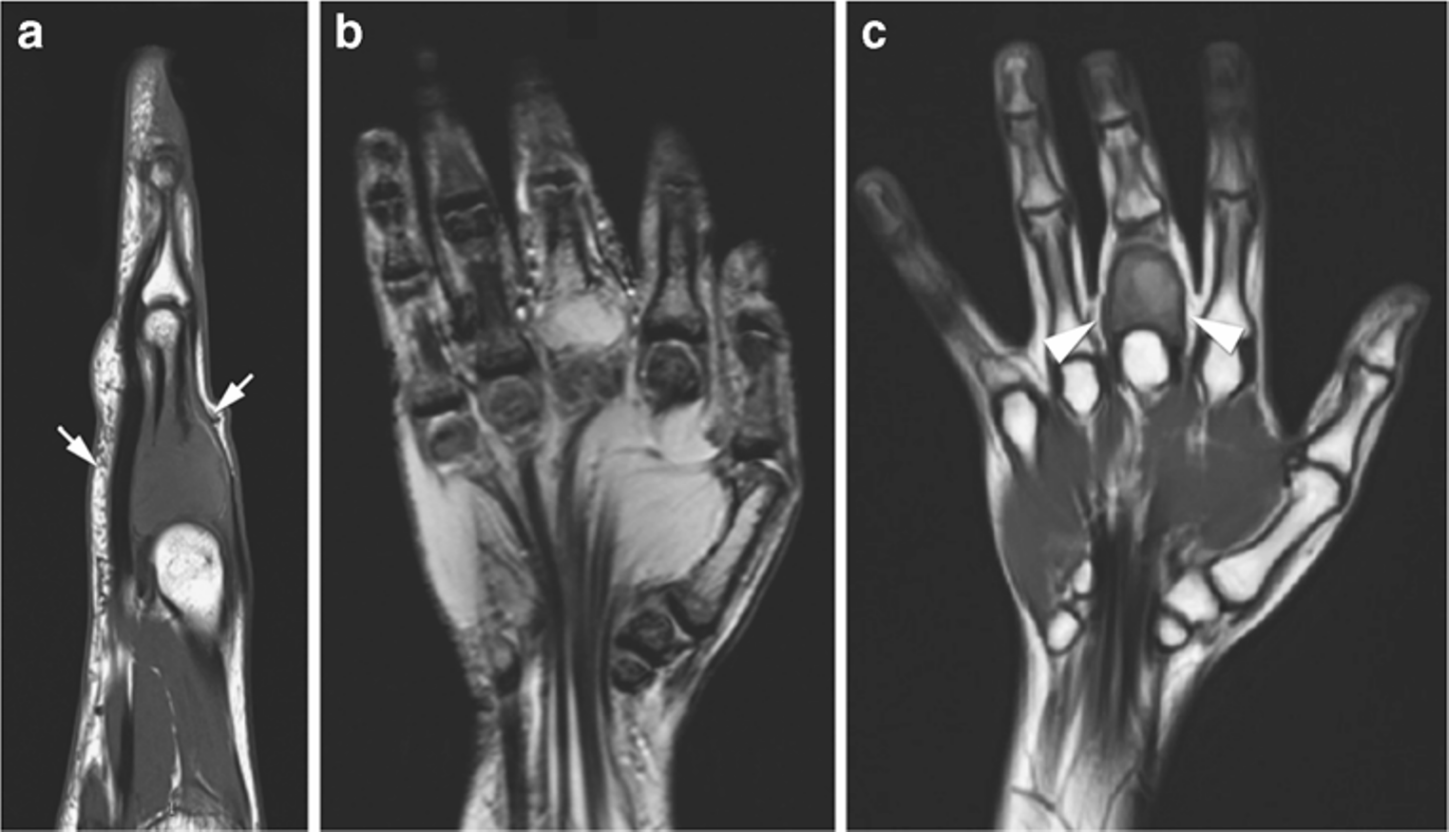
A 17-year-old girl presented with swelling of the middle finger (same patient as Fig. 2). MRI at presentation (a, b) and after 8 weeks on denosumab treatment (c). a Sagittal T1-weighted and b coronal proton density fat-saturated (PD-FS) MR images at presentation demonstrate an expansile, iso-intense T1 and high signal PD-FS GCTB in the proximal phalanx with cortical destruction and soft tissue extension (white arrows). c Coronal T1-weighted MR image after 8 weeks on denosumab shows decreased tumour size and overall lower signal intensity with a low signal intensity sclerotic rim (white arrowheads)
